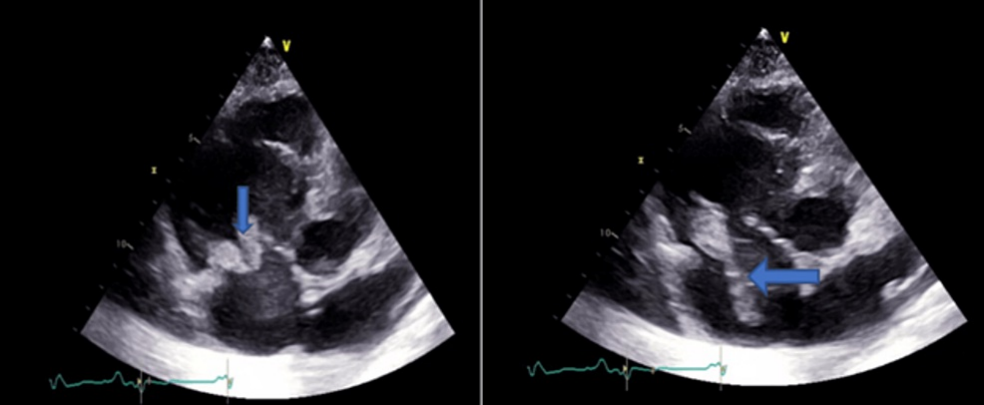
Apical four-chamber echocardiogram. The blue arrows show large and highly mobile vegetations on posterior and septal tricuspid valve leaflets.
